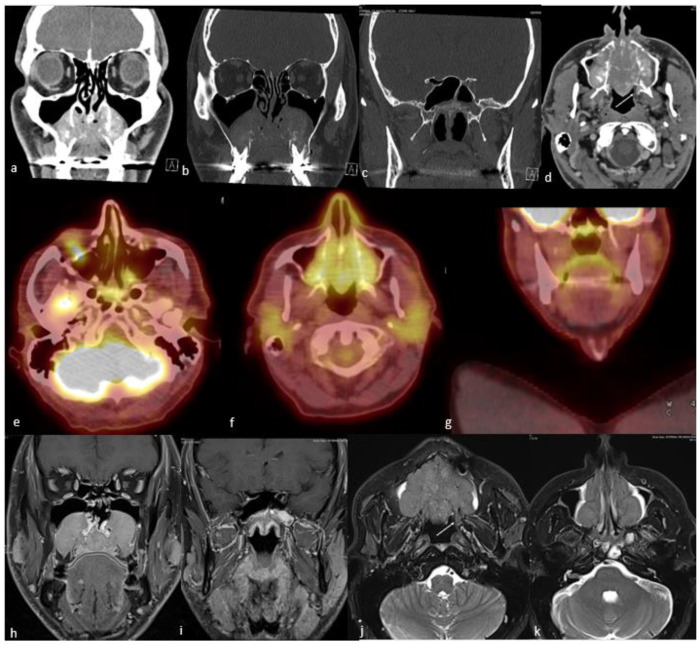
Sinonasal adenoid cystic carcinoma. A 46-year-old male presenting with chronic nasal congestion that had been progressive lately. The patient underwent septoplasty without prior imaging. During surgery, a large bulk mass filling the septal bone was found. Biopsy results were consistent with adenoid cystic carcinoma. CT after septoplasty (a–d) shows an expansile, destructive soft tissue lesion in the nasal cavity, scalloping the hard palate and extending into the bilateral maxillary sinus through erosive changes in the medial maxillary sinus walls. The more posterior coronal image (c) at the sphenoid sinus level shows soft tissue thickening in the floor of the sphenoid sinus with rarefaction of the inferior wall. The axillary slice at the level of the pterygoid plate (d) shows suspicious soft tissue thickening in the left greater palatine canal (arrow). Axial and coronal fused ^18^F-FDG PET/CT images (e–g) show hypermetabolism in the sphenoid floor soft tissue thickening (e,g) and the nasal cavity lesion extending into the maxillary sinuses (f). Post-contrast T1w images (h,i) demonstrate homogeneous enhancement of the nasal cavity lesion extending into the maxillary sinuses (h) and sphenoid floor soft tissue thickening (i). The axial T2w image at the level of the pterygoid plate (j) confirms the CT findings and demonstrates perineural spread along the left greater palatine nerve (arrow). The more cranial T2w image at the level of the pterygopalatine fossa (k) demonstrates normal fat planes without soft tissue thickening, indicating limited perineural tumor spread radiologically.
